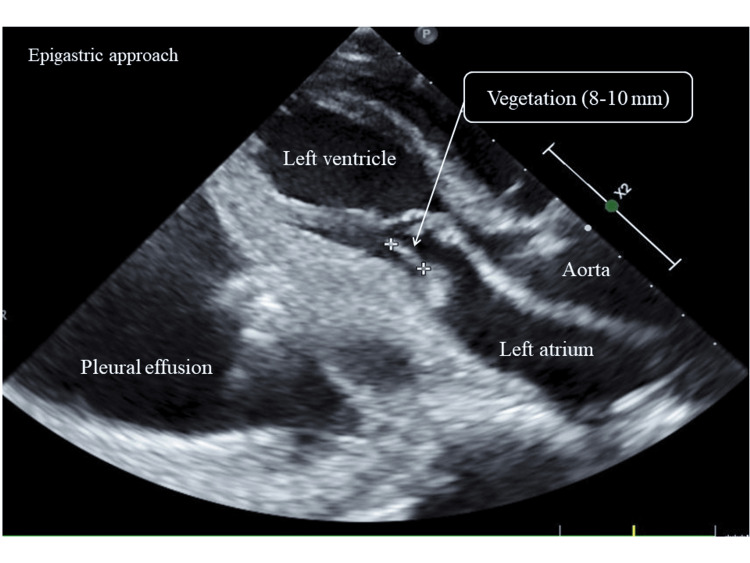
Transthoracic echocardiography on hospital day 16 Transthoracic echocardiography on hospital day 16. This showed vegetation measuring 8–10 mm on the posterior apex of the mitral valve.
